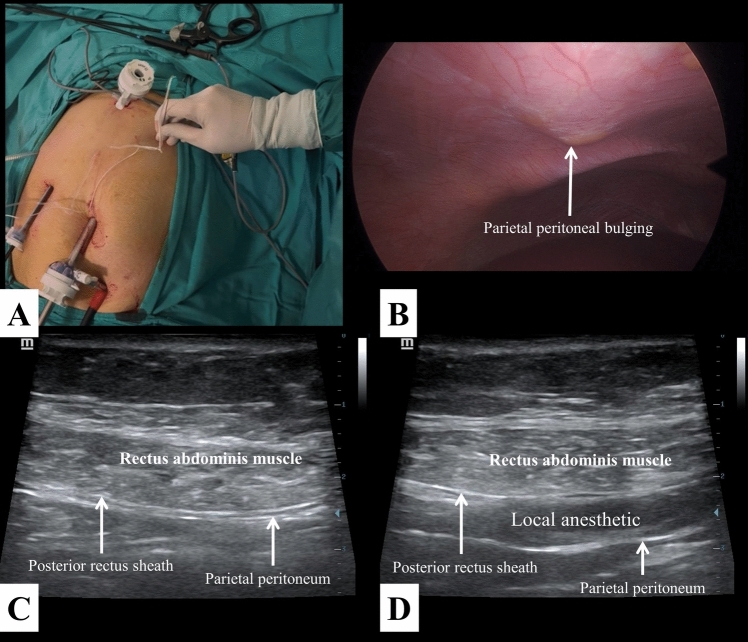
Technique and imaging of laparoscopic-guided deep rectus sheath block. A Intraoperative demonstration of laparoscopic-guided deep rectus sheath block during laparoscopic cholecystectomy. B Laparoscopic view demonstrating convex bulging of the parietal peritoneum following preperitoneal injection of local anesthetic. C Ultrasound image of the anterior abdominal wall before injection showing the rectus abdominis muscle, posterior rectus sheath, and parietal peritoneum. D Ultrasound image after injection demonstrating spread of local anesthetic in the preperitoneal plane deep to the posterior rectus sheath
